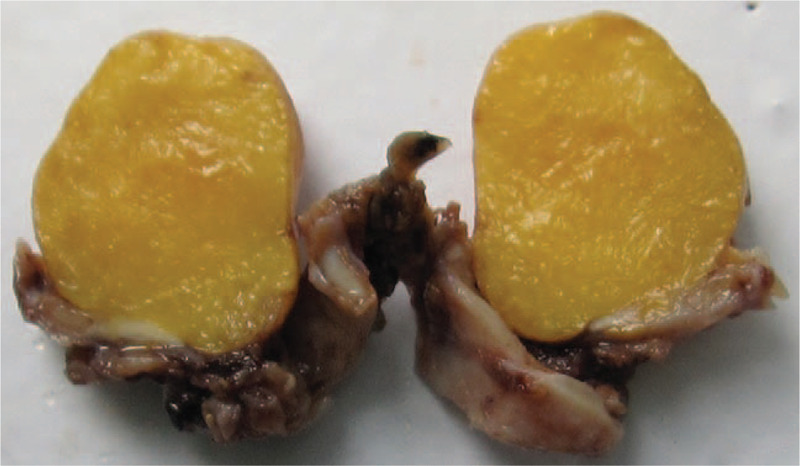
Macroscopic features of the tumor showed a well-circumscribed solid tumor measuring 2.6 × 2.1 × 1.6 cm in maximum dimension. CT: computed tomography.
